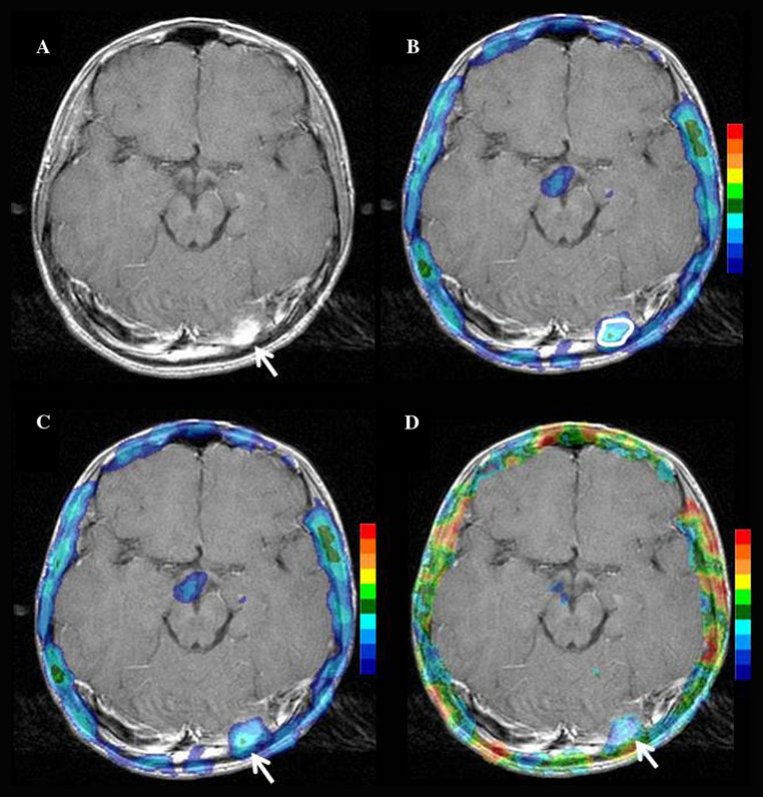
Case 2 is a 13-year-old male patient who presented with a low-grade (grade I) in left occipital pilocytic astrocytoma. a Gadolinium-DTPA enhanced T1 weighted image; b mean UR image; c maximal UR image; d VBA-RI image. Since the tumor existed near the scalp, the MRI was used for reference. The mean and maximal URs of the tumor demonstrated high values of 1.95 and 2.55, respectively, which were suggestive of high-grade glioma. The VBA-RI of the tumor, however, showed a low value of 0.98, which was suggestive of, and in agreement with low-grade glioma
